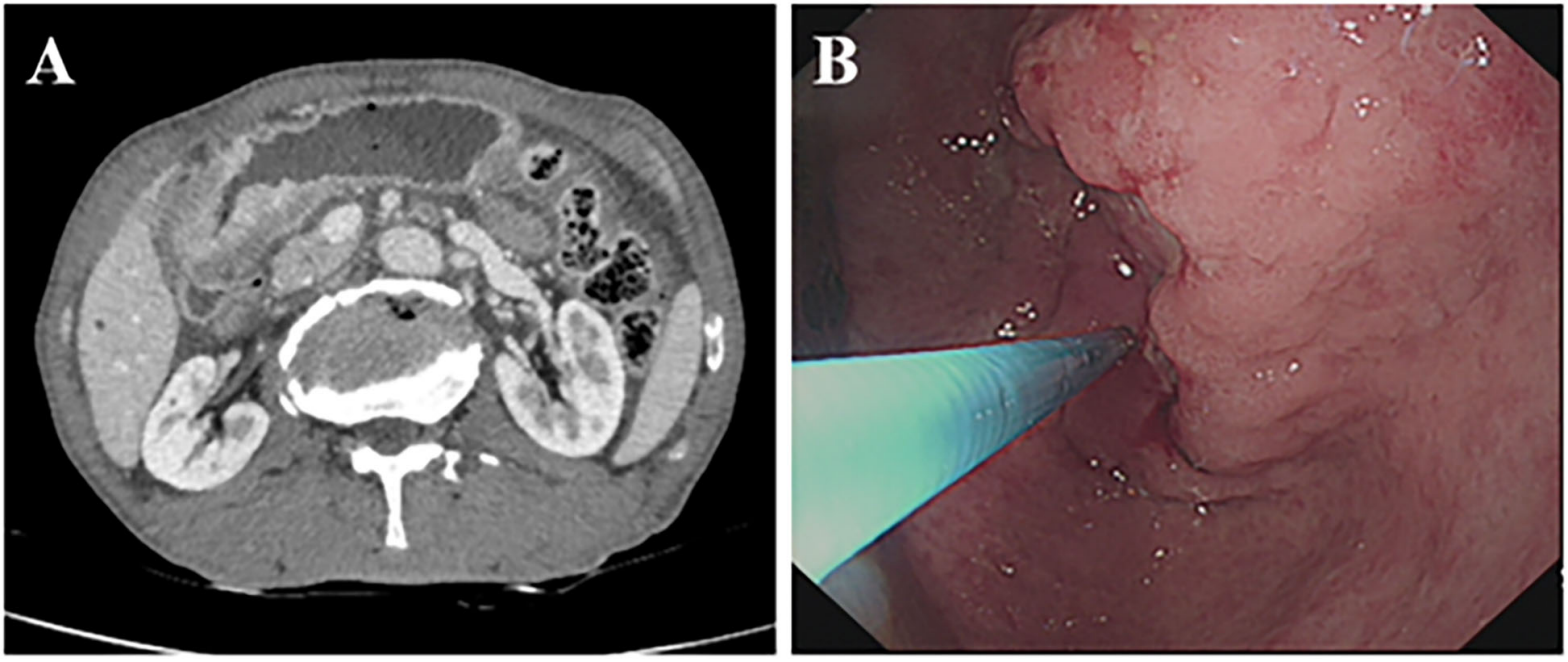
Preoperative imaging and gastroscopy examination. (A) Enhanced CT showing thickening of the gastric wall on the side of the antrum lesser curvature, unclear plasma membrane surface, and obvious enhancement of the mass in the portal stage. (B) Gastroscopy showing irregular ulcer-type lesion in the antrum.
